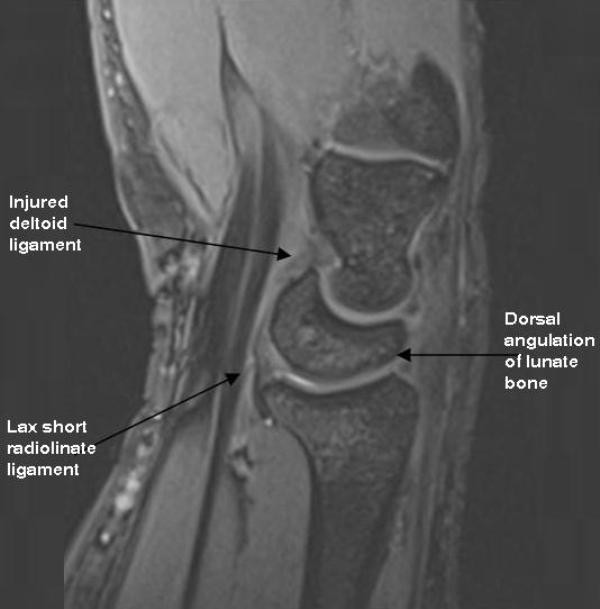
Sagittal MRI image: Ligamentous injuries leading to dorsal angulation of lunate bone - dorsal intercalated segment instability (DISI).
